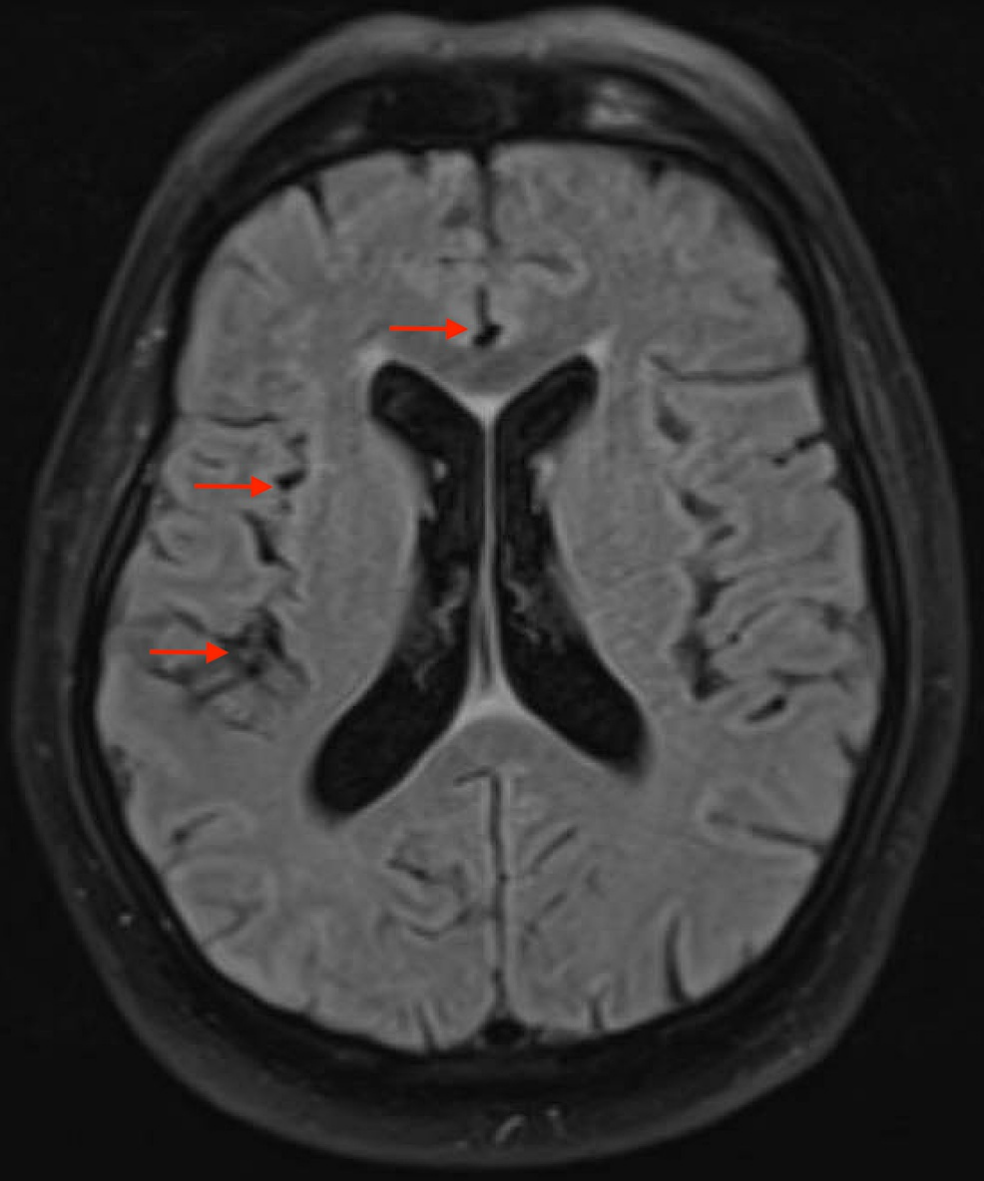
Brain MRI FLAIR axial view Brain MRI fluid attenuation inversion recovery (FLAIR): hypodense area; Red arrows: fat drops; Bright white area: ventricular ependymal enhancement and hyperintense areas.
